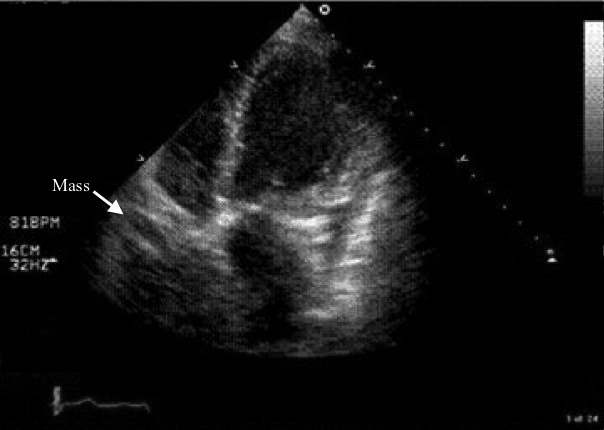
Transthoracic 4 chamber echocardiogram poorly defined pericardial mass overlying right atrial border (arrow) [18]
